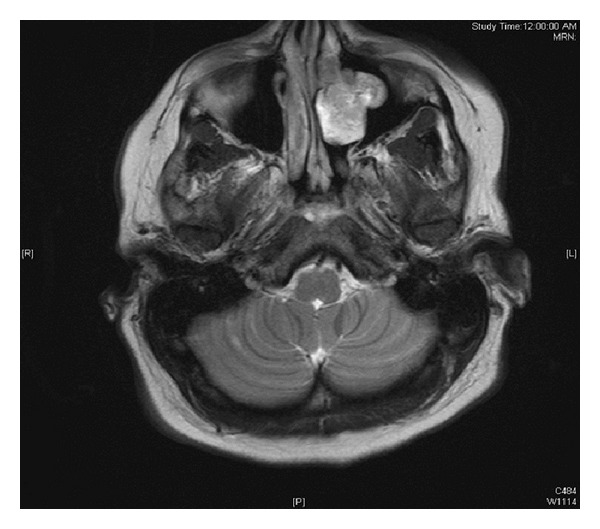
T2 weighted MRI with gadolinium showing enhancing mass in left lateral nasal wall and maxillary sinus.
